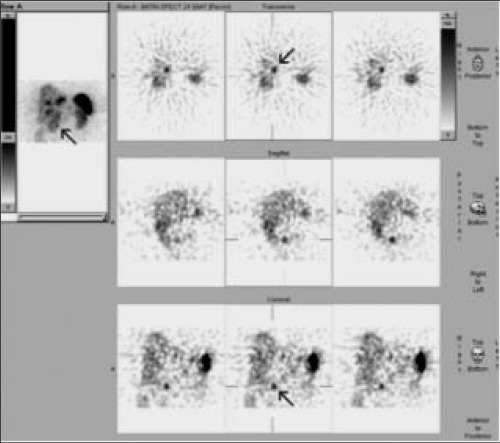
SPECT images at 24 hours show focal tracer uptake at theright side of the upper abdominal region corresponding to duodenumor the uncinate process of pancreas (arrow)
